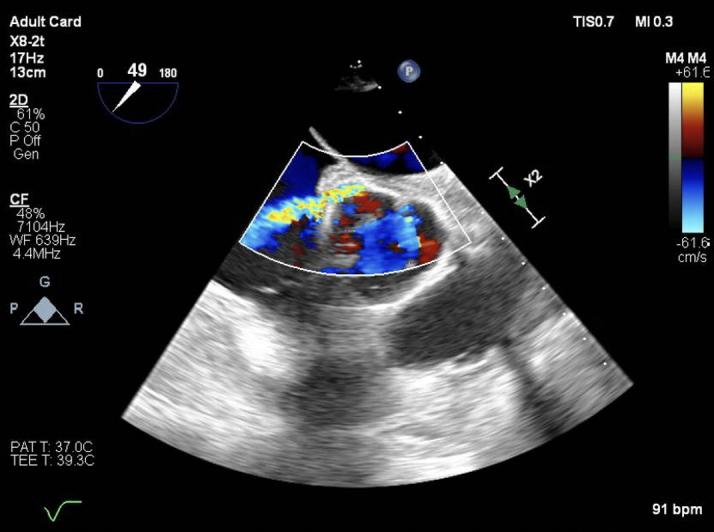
Preprocedural TEE TEE image of a fistula between the noncoronary cusp and the right atrium. TEE = transesophageal echocardiography.
